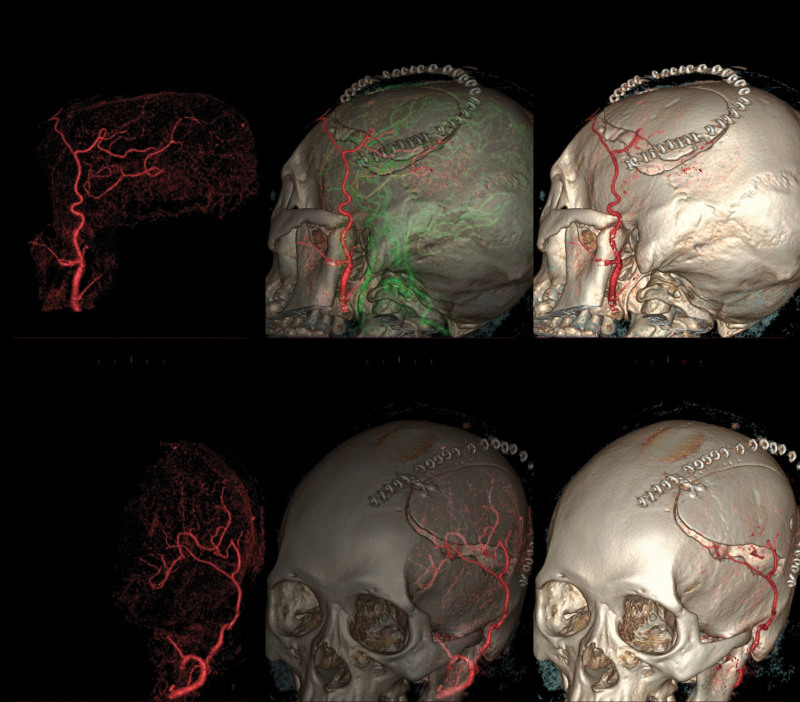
Representative CTA images showing bypass patency in a patient who had undergone left combined bypass surgery. CTA = computed tomography angiography.
